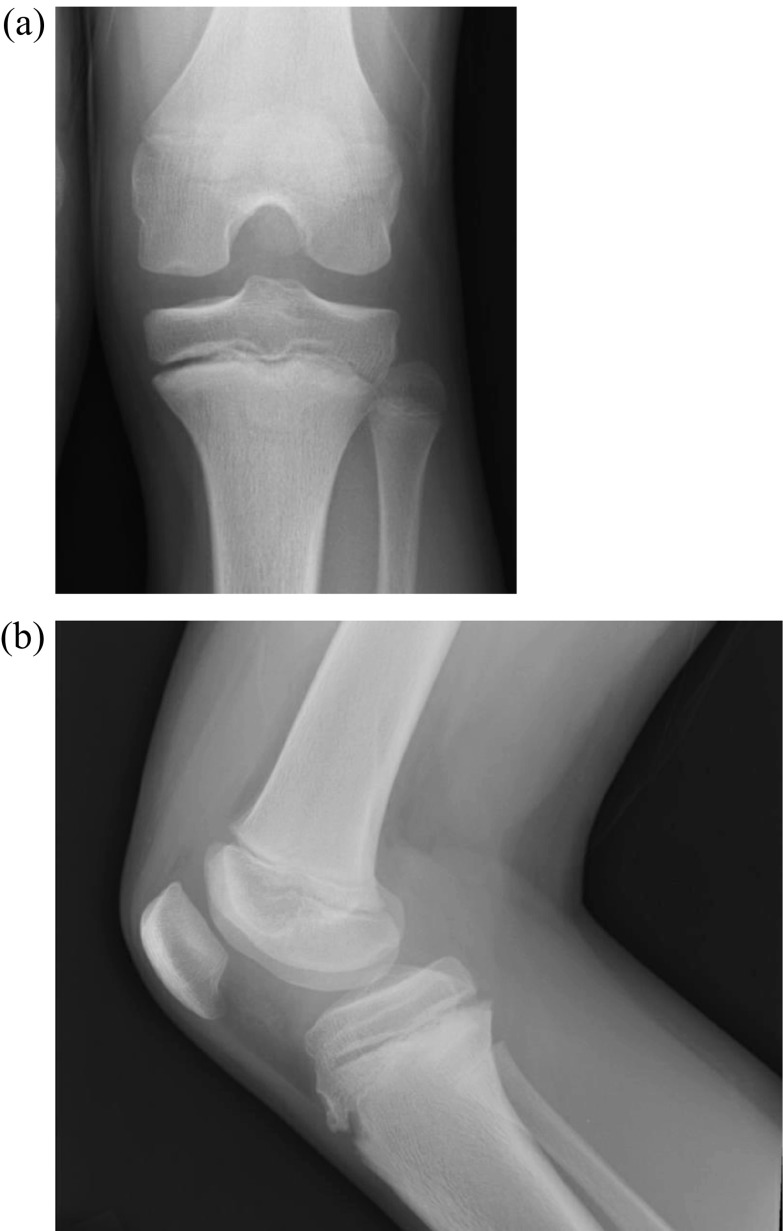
Preoperative AP and lateral radiographs unremarkable for bony pathology (a and b).
